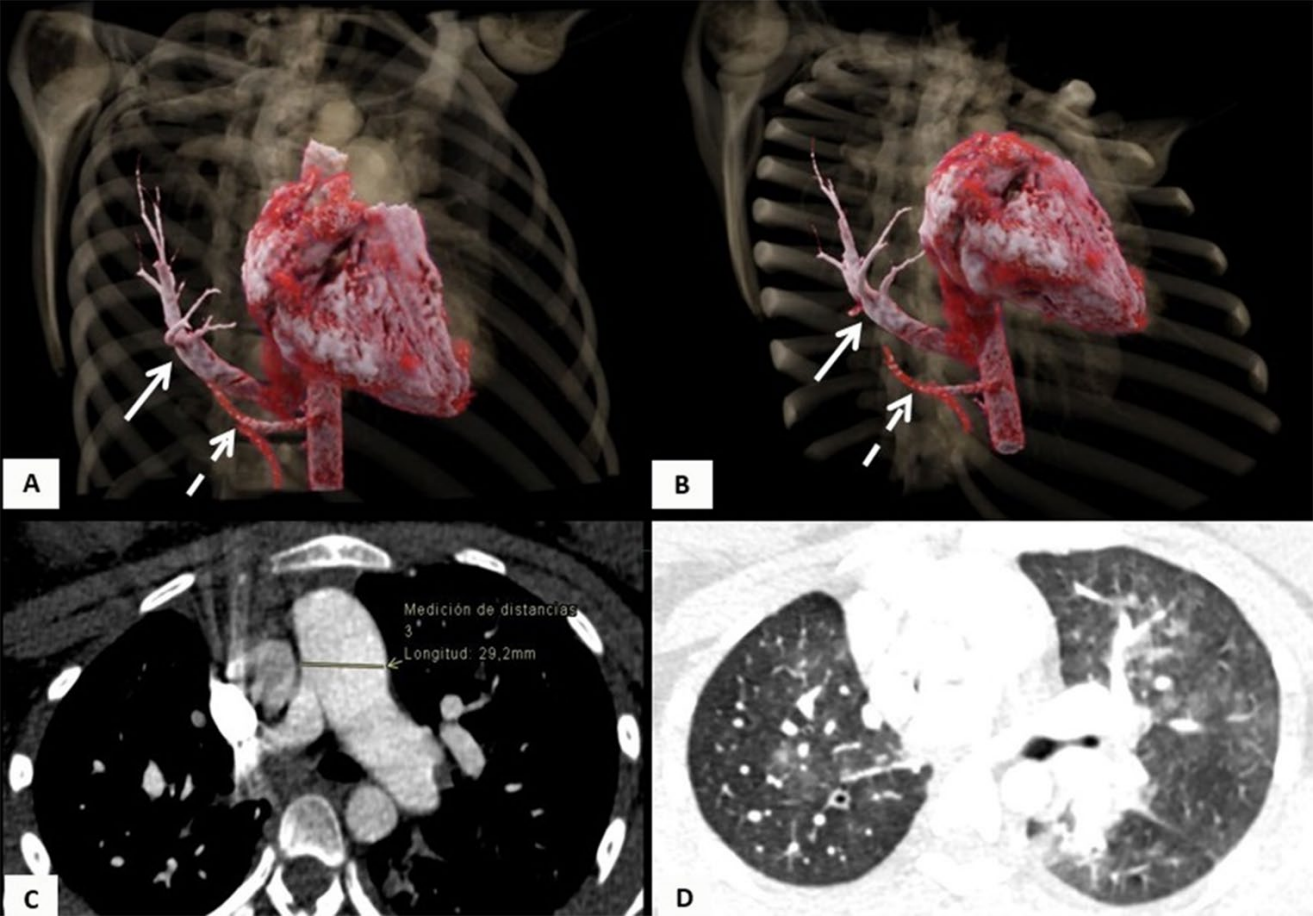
20-year-old female patient with Scimitar syndrome. (A-B) 3D rendered reconstructions of the heart and chest vessels depict anomalous right pulmonary venous drainage into the inferior vena cava (white arrow), also known as Scimitar vein, and an artery originating from the aorta supplying the right lower lobe (Dashed arrow), indicative of a pulmonary sequestration. (C-D) Chest CT angiography shows (C) Increased caliber of the main pulmonary artery (29 mm) and (D) heterogeneous lung parenchyma density due to mosaic perfusion, both signs of pulmonary hypertension
